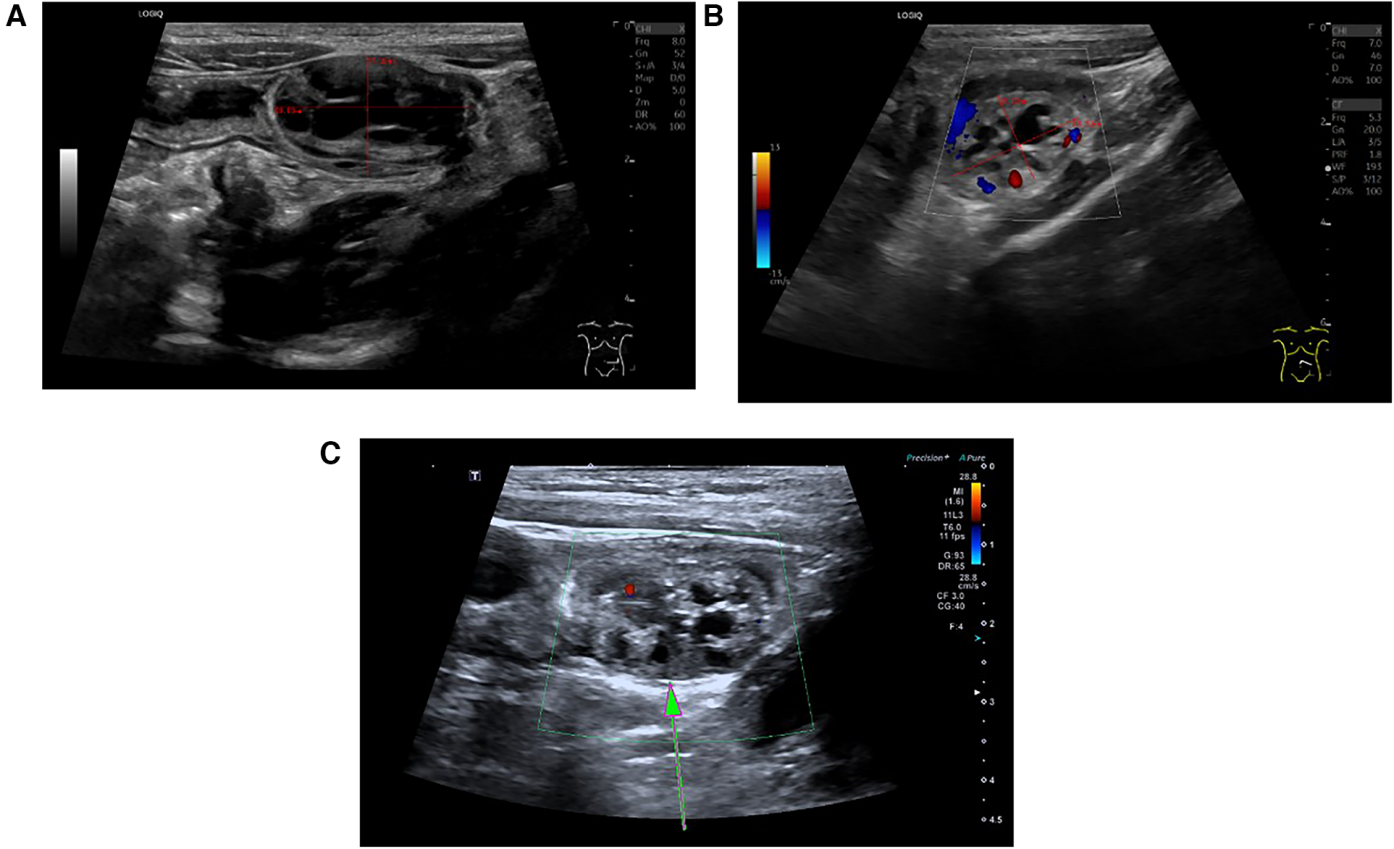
Classic ultrasound image of small intestinal adenomyoma. (A) On the ultrasound image, the small intestinal adenomyoma appears as a honeycomb-like structure, with a size of approximately 28.8 mm × 17.32 mm. (B) Small intestinal adenomyosis presents hypoechogenicity, with punctate or linear blood flow signals displayed within the mass. (C) The area pointed by the arrow is the small intestine adenomyoma.
